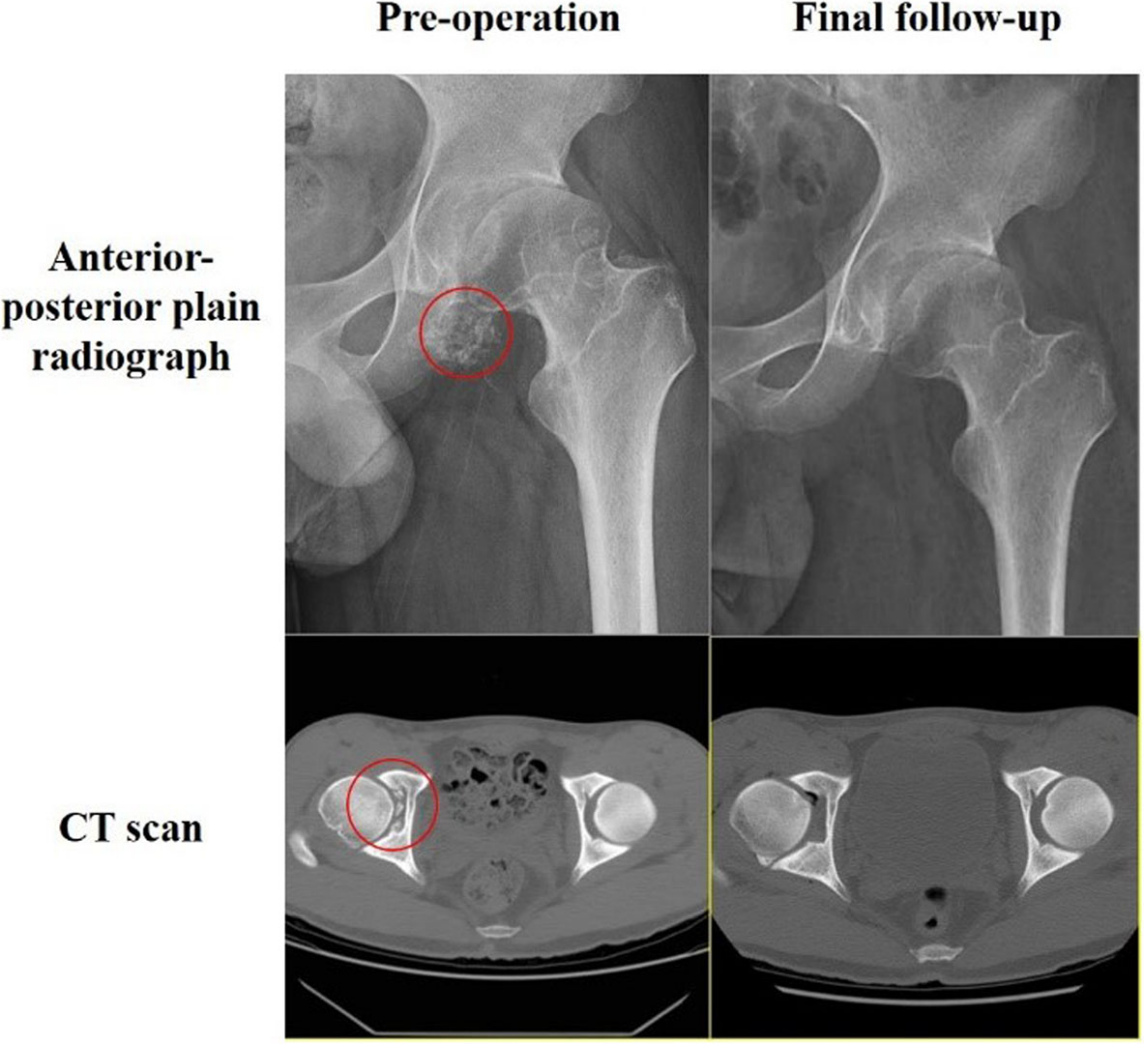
Anterior-posterior plain radiograph and CT scan at pre-operation and the final follow-up. Red circles indicate losse bodies
